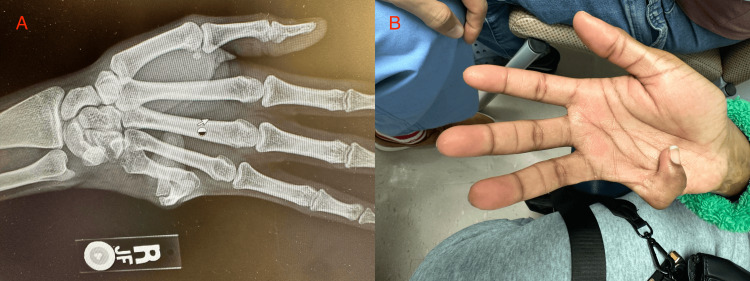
Preoperative evaluation of the right small finger. (A) Preoperative radiograph demonstrating no osseous abnormality. (B) Preoperative clinical photograph (palmar view) demonstrating flexion contracture and visible deformity of the small finger.
